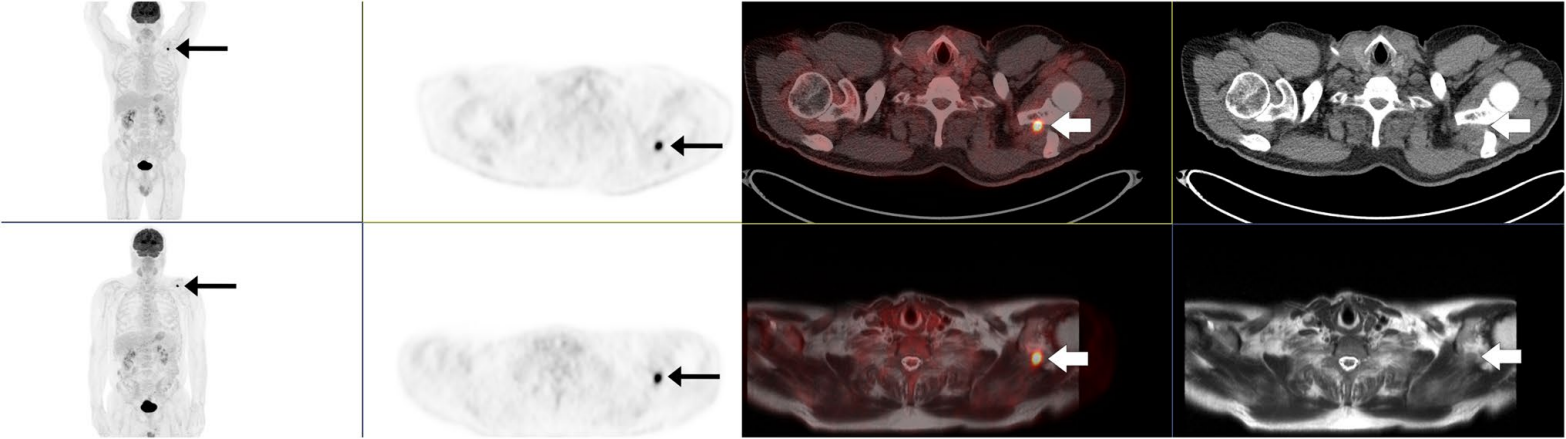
A 71-year-old male with high-grade B-cell lymphoma stage IVA. End of treatment PET/CT in the upper row and PET/MR in the bottom row. The FDG avid lesion was scored as soft tissue on PET/CT and bone marrow on PET/MR. The lesion was localized in scapulae in the MRI sequences T2-HASTE, DWI (b800), ADC map, and T1Dixon in phase. Deauville score 5 and partial metabolic response on both modalities
